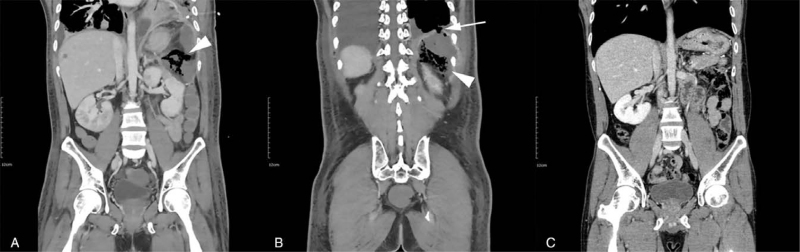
(A), (B) Abdominal CT scan shows perforation of colon at splenic flexure with extraluminal air content (arrowhead) and suspected perforation of left diaphragm with communication with left pleural space (arrow). (C) About 13 mo after emergency laparotomy, abdominal CT scan shows disappeared air bubbles and nearly resolved fluid cavity in left subdiaphragmatic space. CT = computed tomography.
